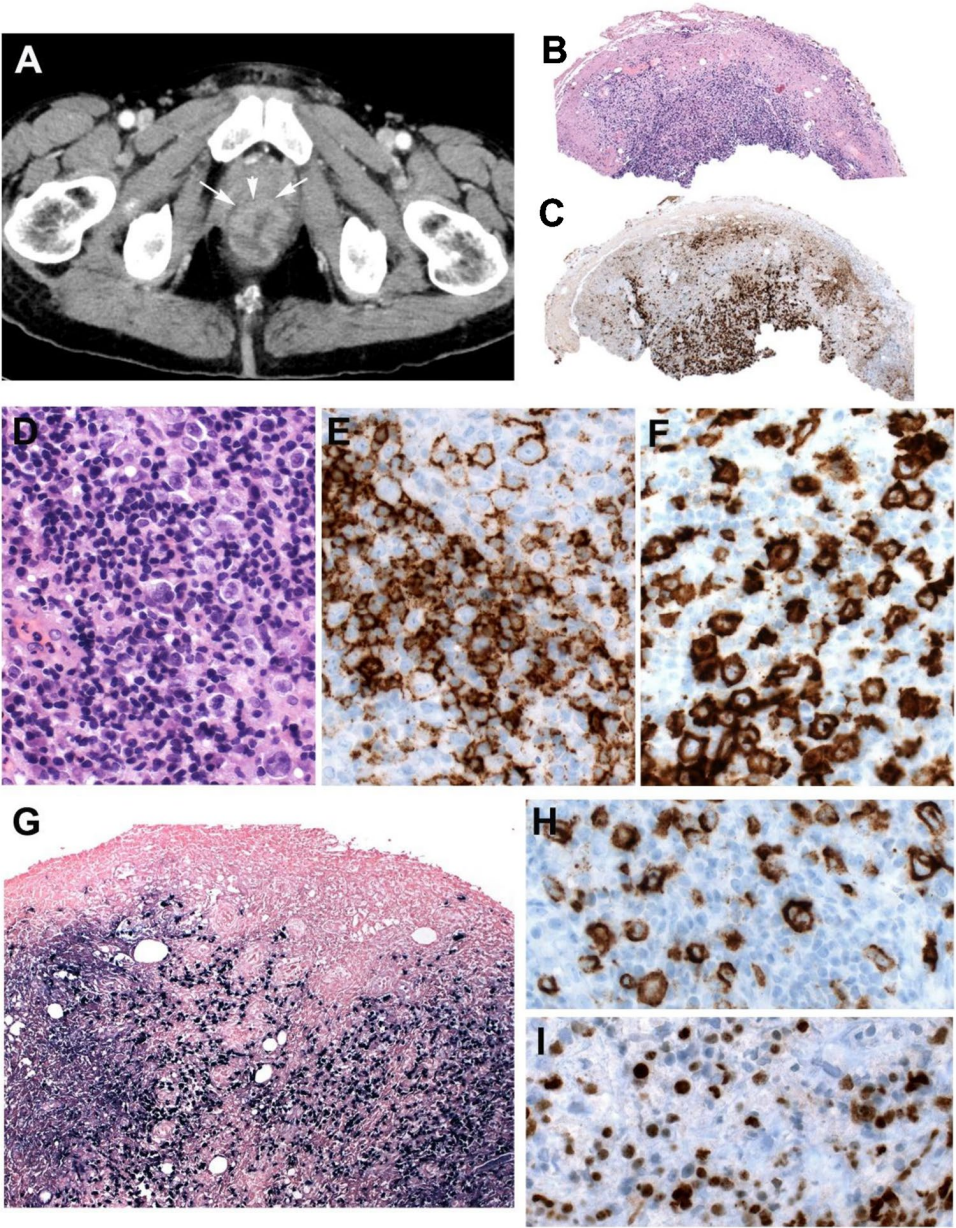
EBV-positive polymorphic B cell lymphoproliferative disorder, NOS. Patient with known Crohn’s disease under azathioprine therapy. A Abdominal CT scan reveals enlarged retroperitoneal lymph nodes and thickening of the rectal intestinal wall (white arrows). B Panoramic view of the rectal biopsy showing an ulcer with a dense polymorphic infiltrate (snap-shot from scanned slide) mimicking an EBV+ mucocutaneous ulcer. C CD3 demonstrates a rim of T cells at the base of the ulcer (snap-shot from scanned slide). D Higher magnification demonstrates a polymorphous infiltrate with Hodgkin and Reed–Sternberg (HRS)-like cells. E CD20 is positive in the large atypical cells, as well as in the small lymphocytes. F CD30 is positive in the B cell infiltrate. G Low-power view of the ulcer with abundant EBER-positive cells (original magnification, ×50). The cells are LMP1-positive (H) and EBNA2-positive (I) revealing an EBV latency 3. (D–F and H–I original magnification, ×400)
